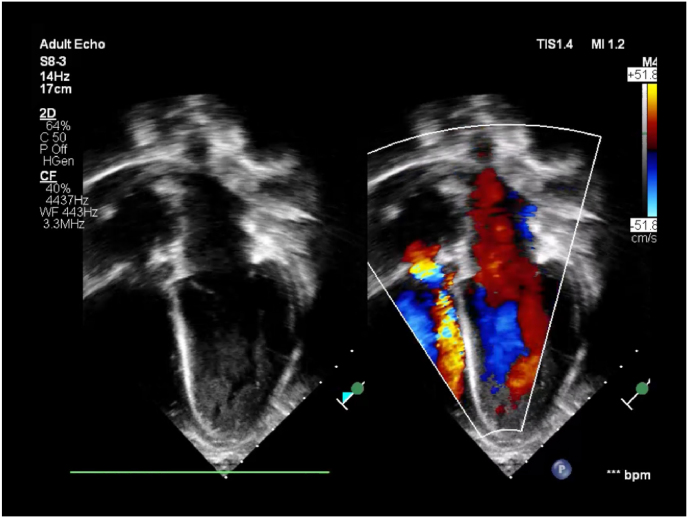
Postoperative follow up echocardiogram showing normal cardiac anatomy. Tricuspid bioprosthetic valve is well positioned with mild stenosis and mild regurgitation. Right ventricular function is mildly to moderately reduced. Trace pericardial effusion is present.
